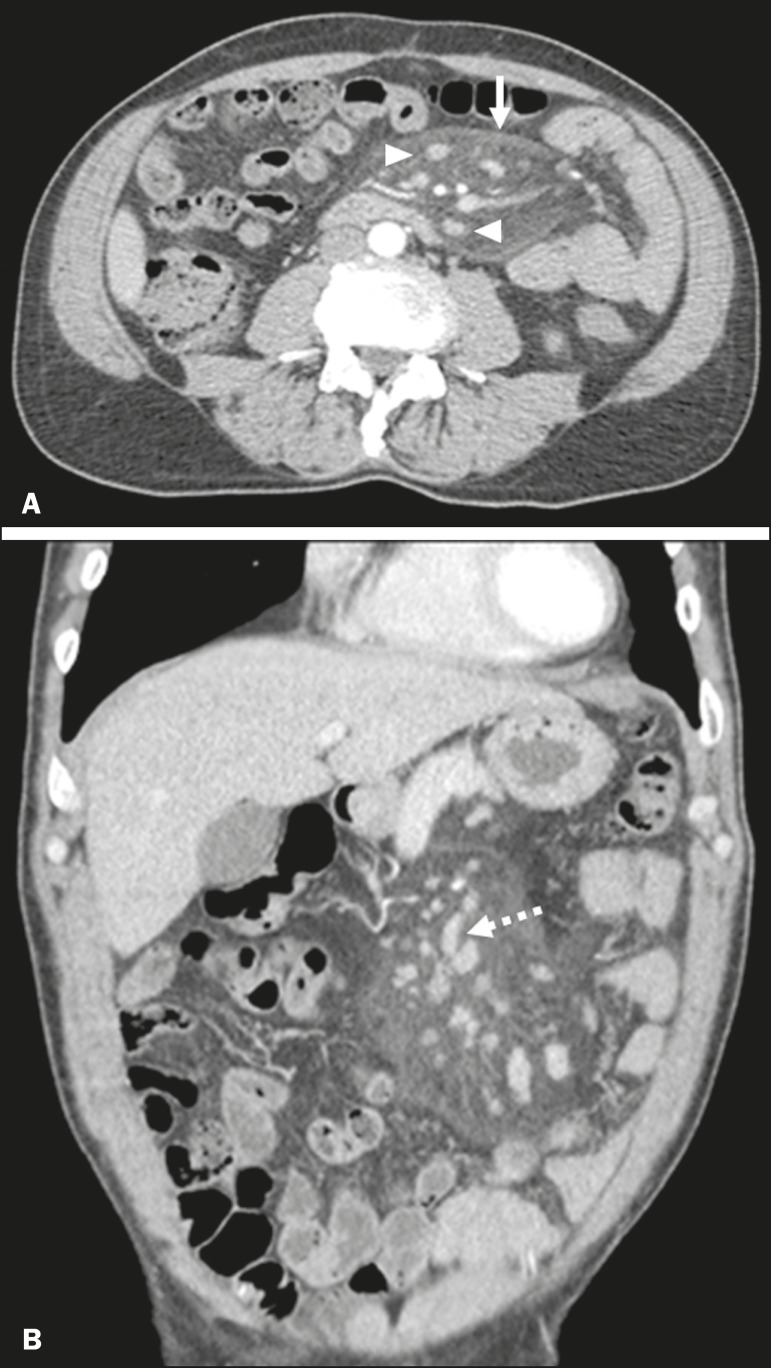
Axial and coronal CT (A and B, respectively)
